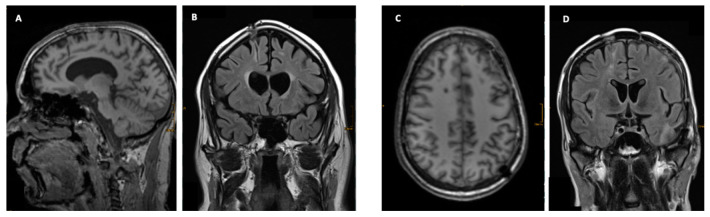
Examples of two patients without a lesion, but with a visible trajectory after probe removal. Panel (A,C) present T1-weighted MRI with corresponding coronal imaging (Panel (B,D)).
